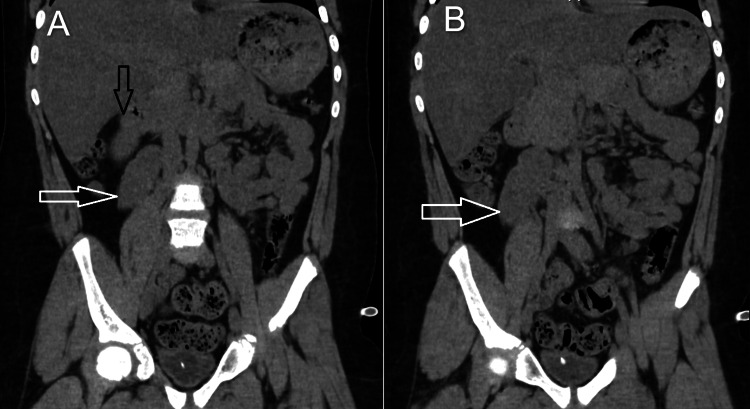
Coronal CT. A) white arrow shows a left ectopic kidney with no gross signs of fusion, and the black arrow is the normal right kidney. B) shows a left ectopic kidney (white arrow)
